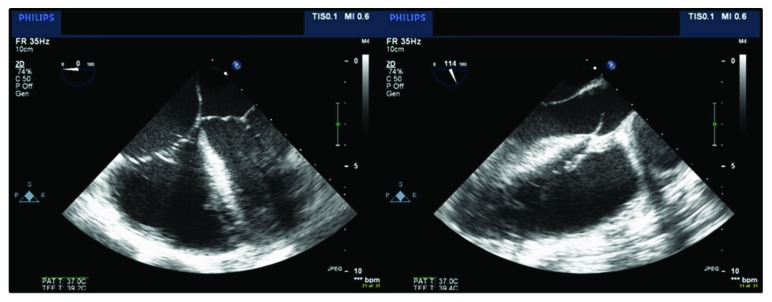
Intraoperative transesophageal echocardiogram. D-shaped ventricle indicating right heart strain seen in multiple frames.
